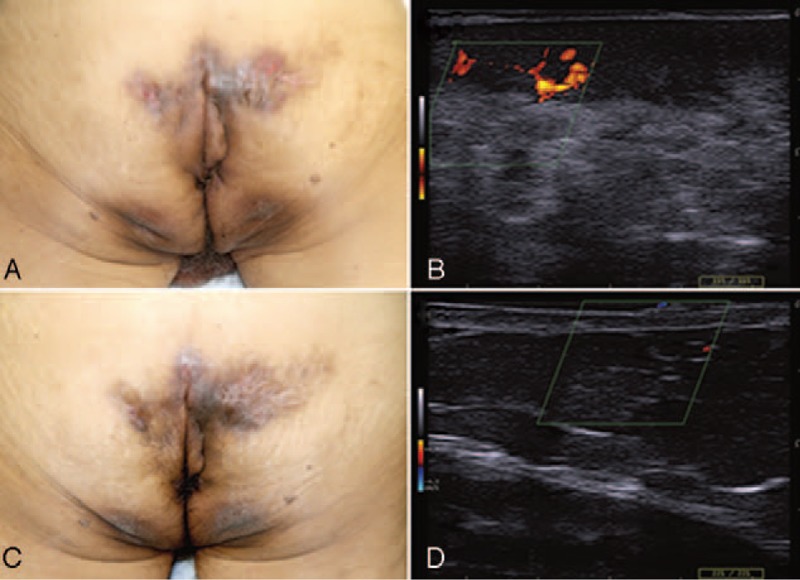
Case 1: (A) Severe Hurley III-hidradenitis suppurativa (HS-PGA 4/5) characterized by complex sinus-tracts and abscesses. (B) Doppler ultrasound examination showing inflamed tunnels on the right buttock, at baseline. (C) Clinical improvement after 16 weeks of apremilast treatment. (D) Decreased inflammation/color-flow signal after 16 weeks of treatment.
